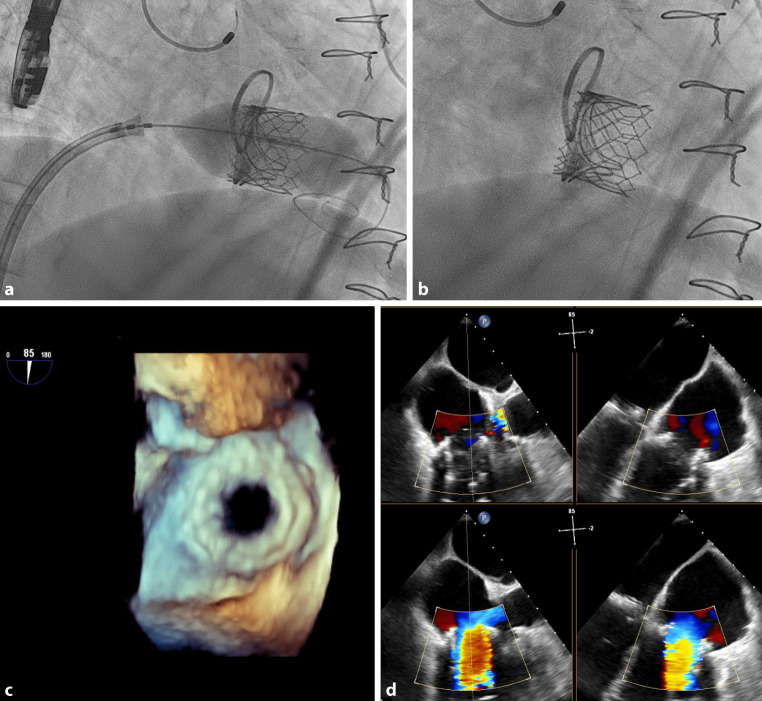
Fluoroscopy of the balloon implantation (a, supplemental video 3) and the final result (b, supplemental video 4), postprocedural early diastolic image using echocardiography, the stent of the transcatheter valve is visible (c, supplemental video 5), postprocedural biplane imaging midsystolic (d, *upper images*, supplemental video 6) and early diastolic (d, *lower images*, Supplemental video 6)
